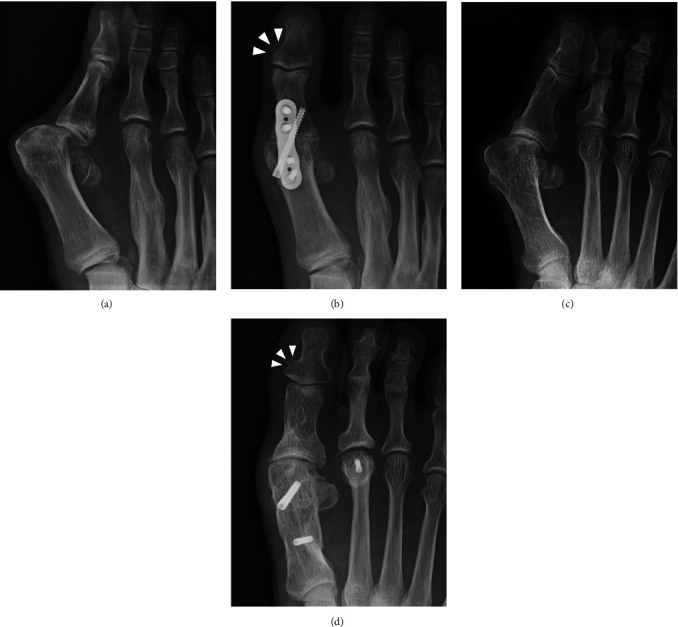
Representative anteroposterior foot radiographs of the cases showing increased width of medial bone excrescence at the distal phalanx of the hallux after the metatarsophalangeal arthrodesis (a, b) or metatarsal osteotomy (c, d) performed to rectify hallux valgus deformity. Arrowheads indicate the protruded bone excrescence after the procedures.
